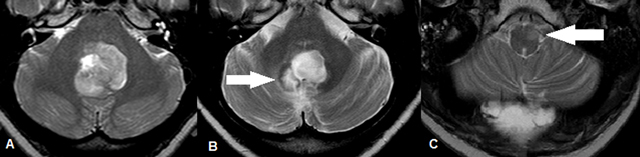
Initial MRI demonstrates a mass in the fossa posterior and fourth ventricle with heterogeneous high signal intensity on axial T2-weighted images and only moderate enhancement after injection of intravenous gadolinium (not shown) (A). MRI obtained seven months postoperatively. The axial T2-weighted image on the left shows a postoperative defect in the right dentate nucleus (white arrow) (B) and a new expansile T2- hyperintense lesion in the left inferior olivary nucleus (white arrow) (C).
